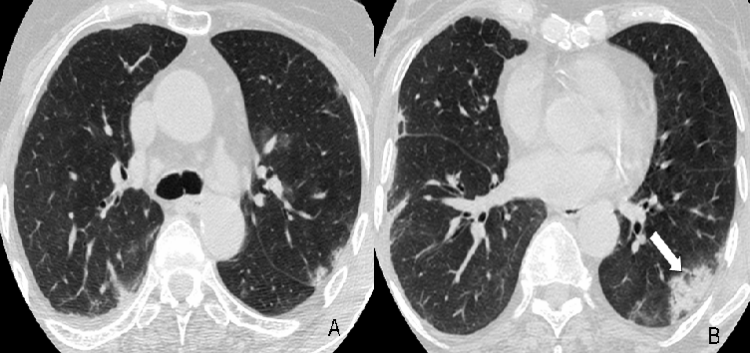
High-resolution computed tomography (HRCT) after three months of continuous corticosteroid therapy at the level of the carina (A) and lower lobes (B) at lung windowing shows significant improvement with almost complete resolution of most of the previously seen consolidations. However new small "migratory" subpleural and peribronchovascular opacities are seen in the left lower lobe (arrow) in a previously uninvolved area.
